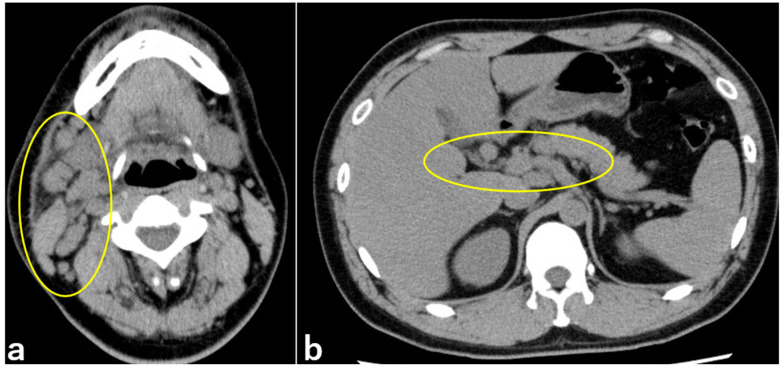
Computed tomography taken during the initial visit to our department. Lymphadenopathy (circled in yellow) was observed in the right parotid gland (a) and hilar region (b).
